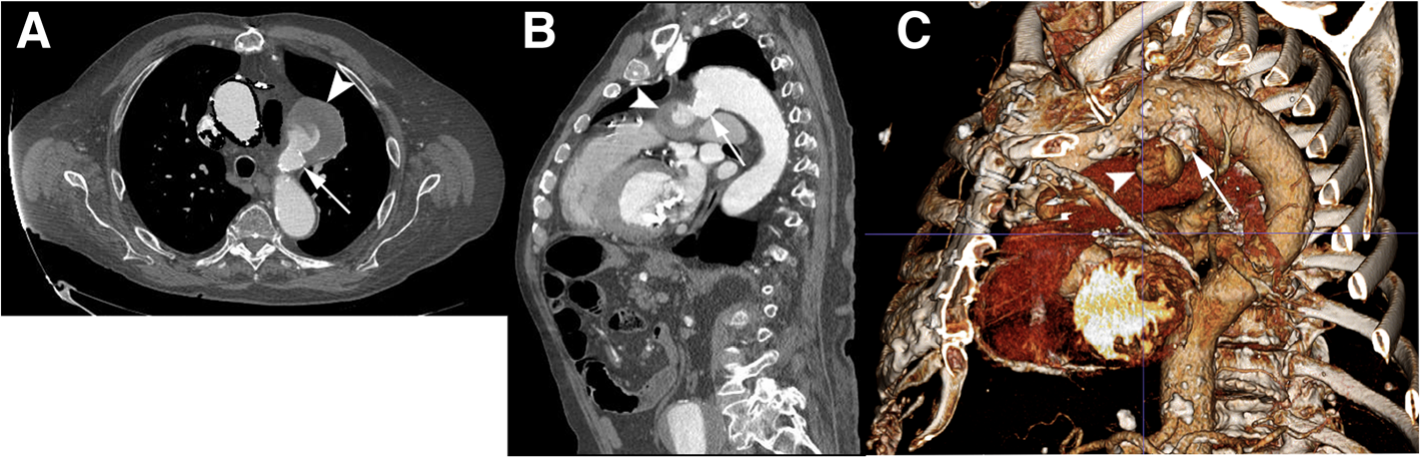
Contrast-enhanced CTA of the thoracic aorta showing the ductus arteriosus diverticulum (DAD) (arrow) and pseudoaneurysm (arrowhead) a Axial image demonstrates junction of DAD and pseudoaneurysm b Two-dimensional reformatted sagittal plane image delineates the relationship between the aorta, the DAD, and the pseudoaneurysm. c Three-dimensional reconstruction of thoracic aorta for visualization and surgical planning
